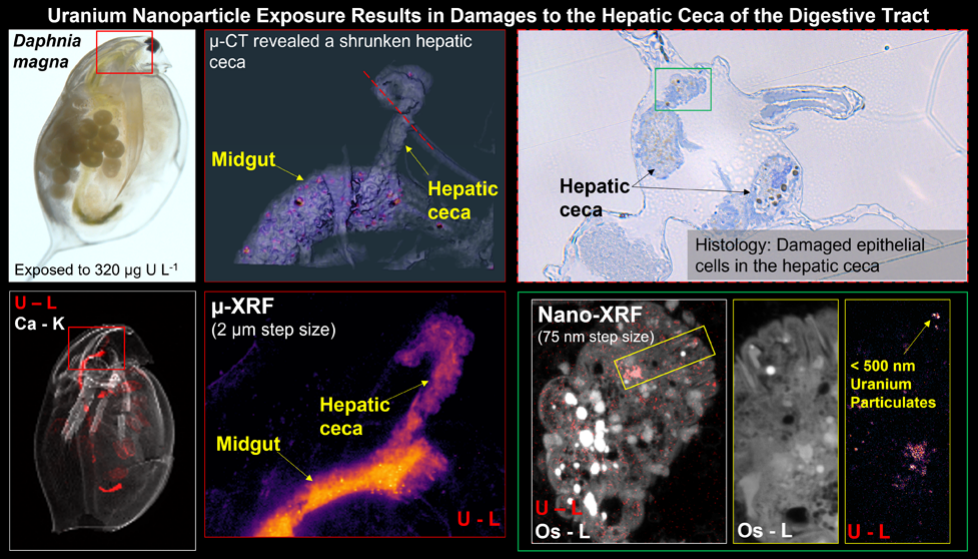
Micro- and nanoscopic X-ray techniques were used to investigate
